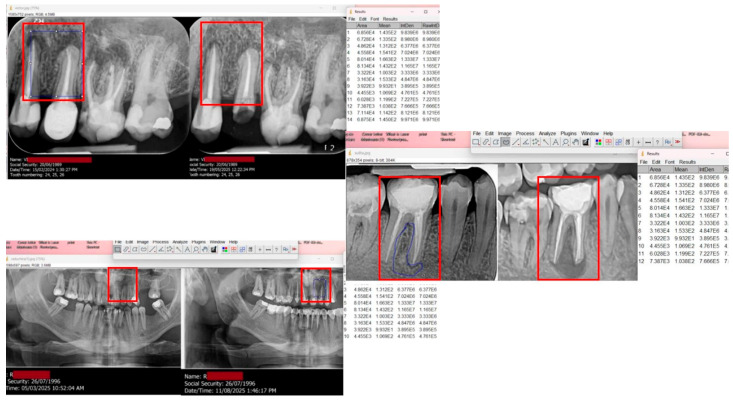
Periapical radiographs of maxillary anterior teeth showing bone density measurements using ImageJ software. The left panel shows the baseline radiograph, and the right panel displays the follow-up image after treatment. The rectangular region of interest (ROI) on the right image indicates the area selected for grey-scale density analysis. Quantitative results, including area, mean grey value, and integrated density, are presented in the Results window to the right.
